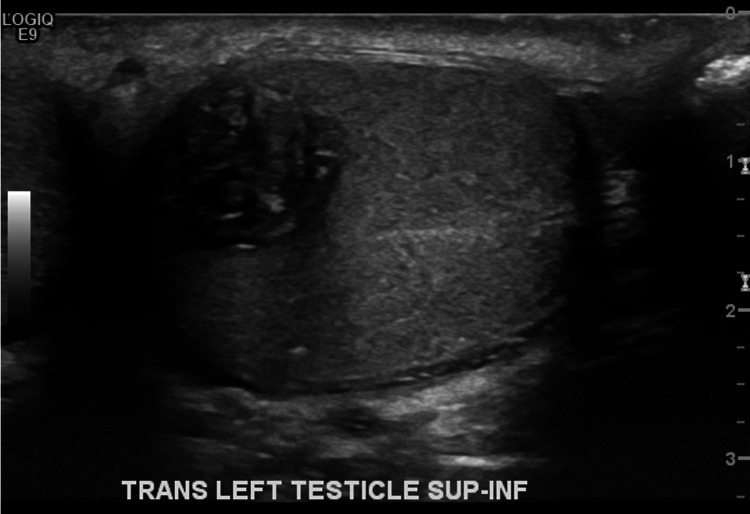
Left Testicular Mass on Ultrasound
